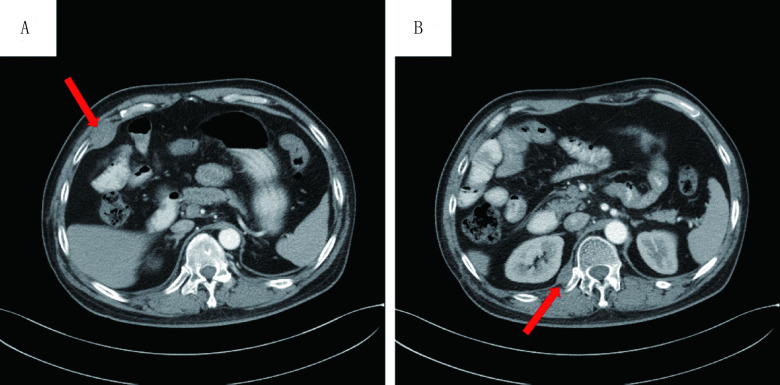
口服阿帕替尼治疗后的CT扫描图像。2017年12月CT扫描显示病情进展（A、B）。 Chest CT scans after taking apatinib. December 2017 CT scans showed that progression disease (A, B).
